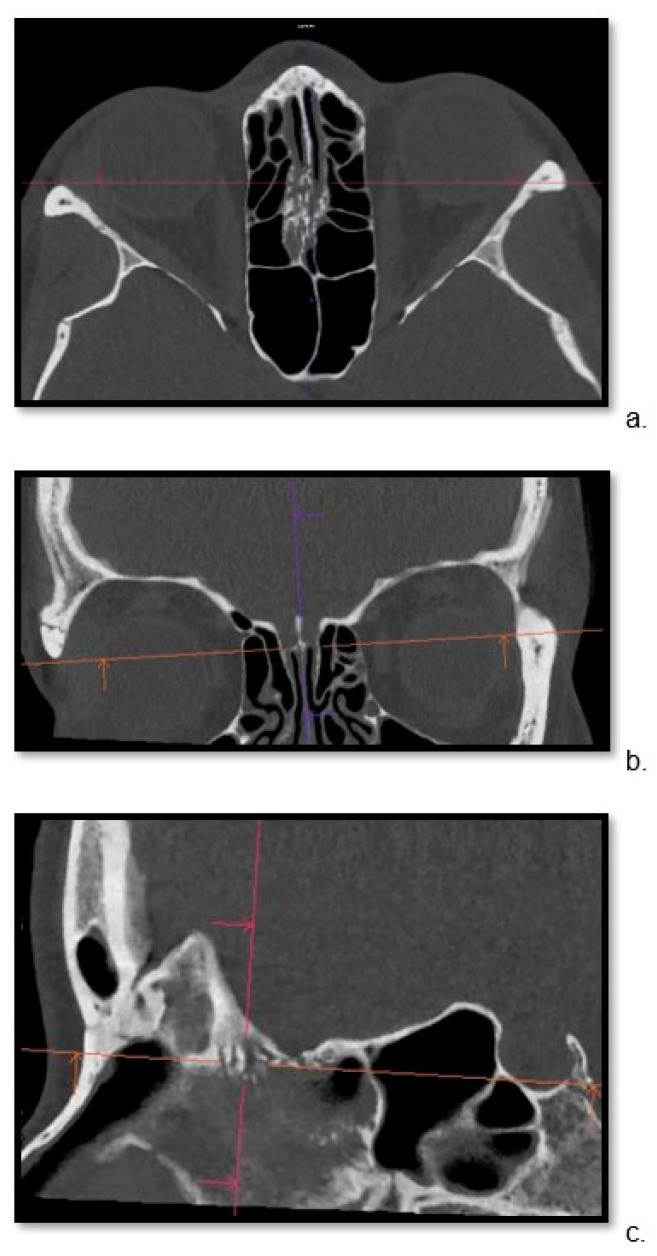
Three-dimensional localization of cribriform plate. Thin-layer imaging of the ethmoidal crest using photon-counting computed tomography. Examination in multiplanar reformation includes (a) axial, (b) coronal, and (c) sagittal views on thin-layer CT.
